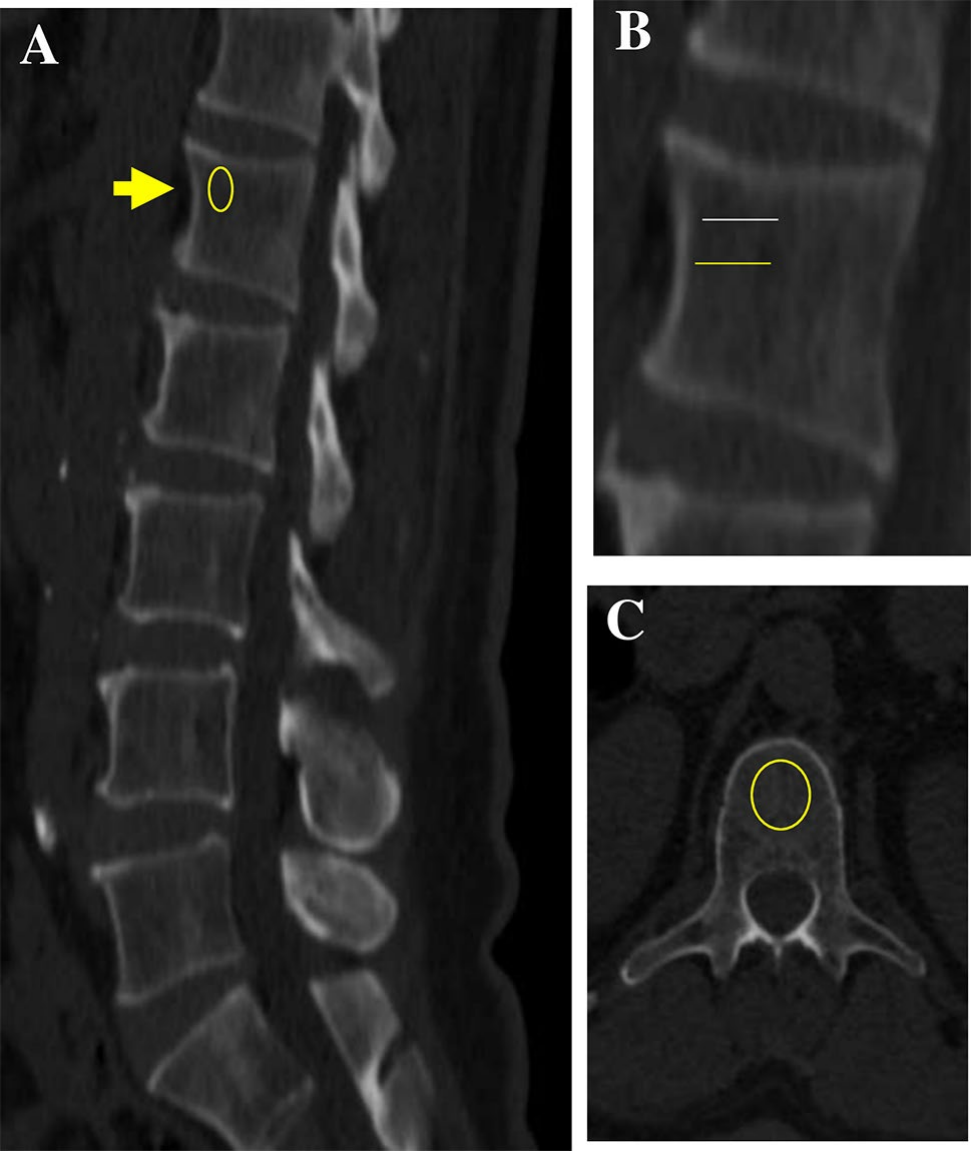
Example of unenhanced computed tomography (CT) images of the spine of a 59-year-old woman. (A) Bone window showing the first lumbar vertebra level (arrow) and oval yellow ring shows the ROI, (B) Magnified view of the first lumbar vertebra and region of interest. The yellow midline line matches the automated level; the white line reflects the standard manual level. (C) First lumbar vertebra axial image; oval yellow ring shows the region of interest. CT examination was performed at a constant peak voltage of 120 kV.
